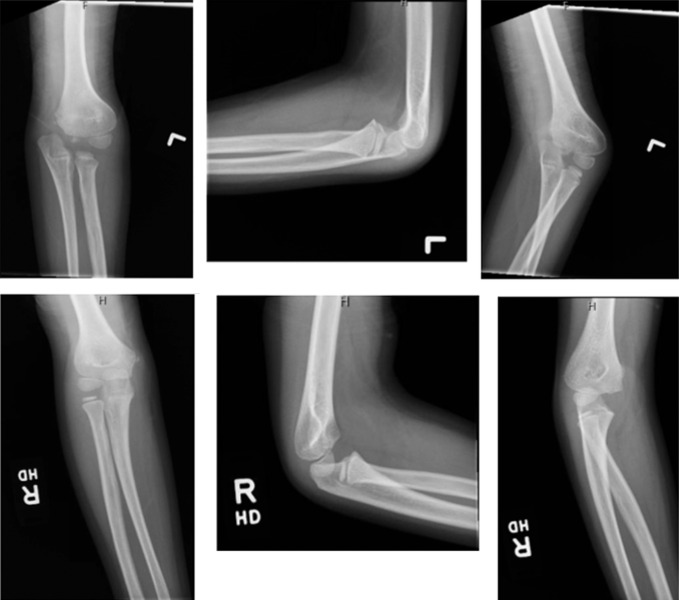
Radiographs demonstrating the AP, lateral, and oblique x-rays of the left and right (unaffected) elbows 4 years after the initial injury. The left elbow films show the lack of congruence at the ulnohumeral articulation because of the collapse of the articular cartilage.
